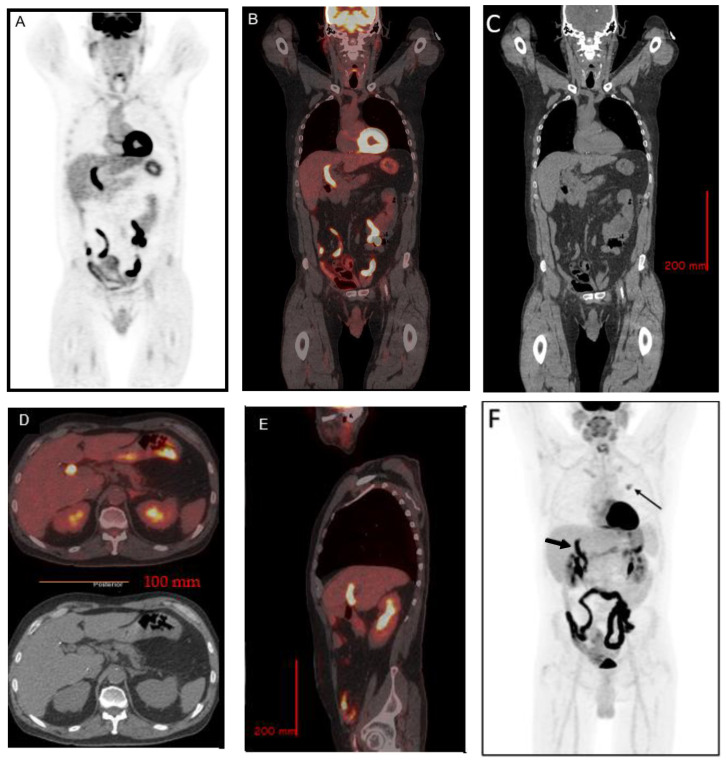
Intense ^18^F-FDG uptake (SUV_max_ = 13.3) along the CBD (coronal images, (A). PET, (B). PET/CT, (C). CT, (D). Trans-axial images, PET/CT and CT, (E). Sagittal images, PET/CT and (F). Maximum intensity projection, thought to be due to ERCP-induced inflammation. There is mild fat stranding adjacent to the CBD on the corresponding low-dose CT images without a definite radiopaque stone in the distal CBD. On MIP, intense uptake at common bile duct is noted (short arrow). There is a hypermetabolic lesion in the left lung (long arrow), confirmed to be a metastasis from colon cancer on biopsy. The patient has a remote history of colon cancer 26 years ago, status post-colectomy with ileostomy. Intense uptake in the bowel loops is secondary to metformin use.
